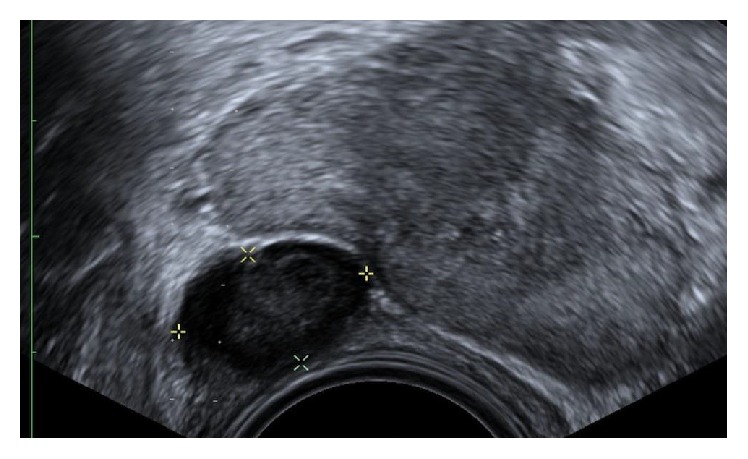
Transrectal ultrasound of the prostate gland. Image taken before core biopsy demonstrates a well-circumscribed lesion on the right, which is more convincingly extracapsular to the prostate gland and contains echogenic internal echoes (callipers).
